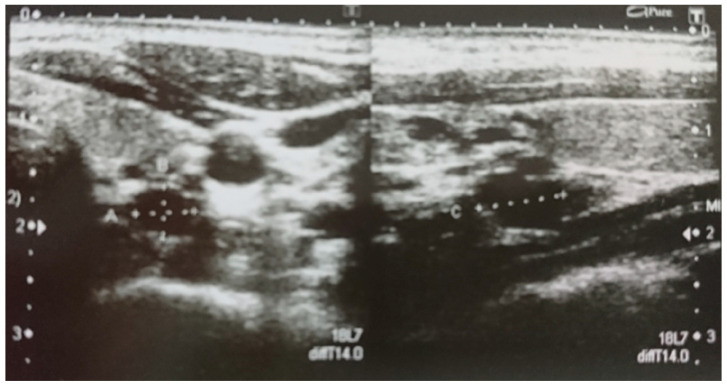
Sonographic image of left-inferior parathyroid adenoma before the 1st surgery.
